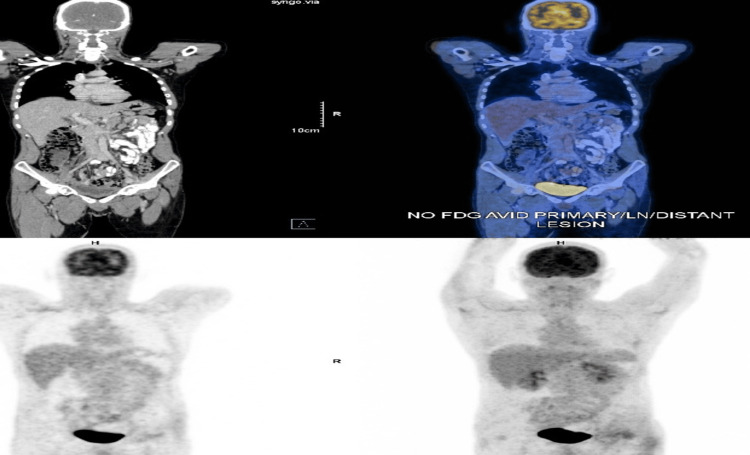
Whole-body FDG PET-CT demonstrating no metabolically active lesions. FDG PET-CT: fluorodeoxyglucose positron emission tomography-computed tomography
